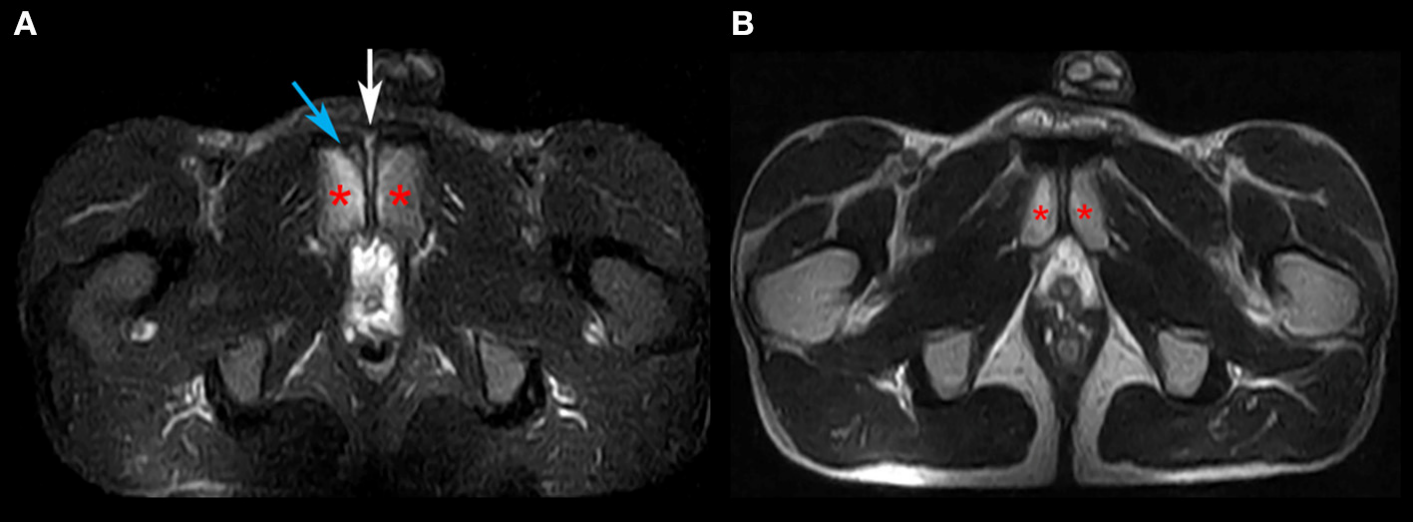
MRI investigation on the axial plane. (A) STIR. (B) T2W. Images highlight the irregularity of the superficial cortical profile identifying osteochondral dissociation of the area (blue arrow), with coexistent endo-articular hyperemia (white arrow) and light subchondral bone hyperemia (left). The pubis is marked with an asterisk.
